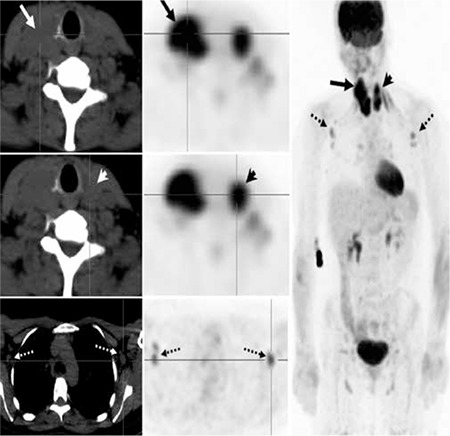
Axial image from neck CT demonstrated asymmetrically enlarged thyroid gland (right left) with lobulated appearance and mild compression of the right side of the trachea (Solid white arrow).
